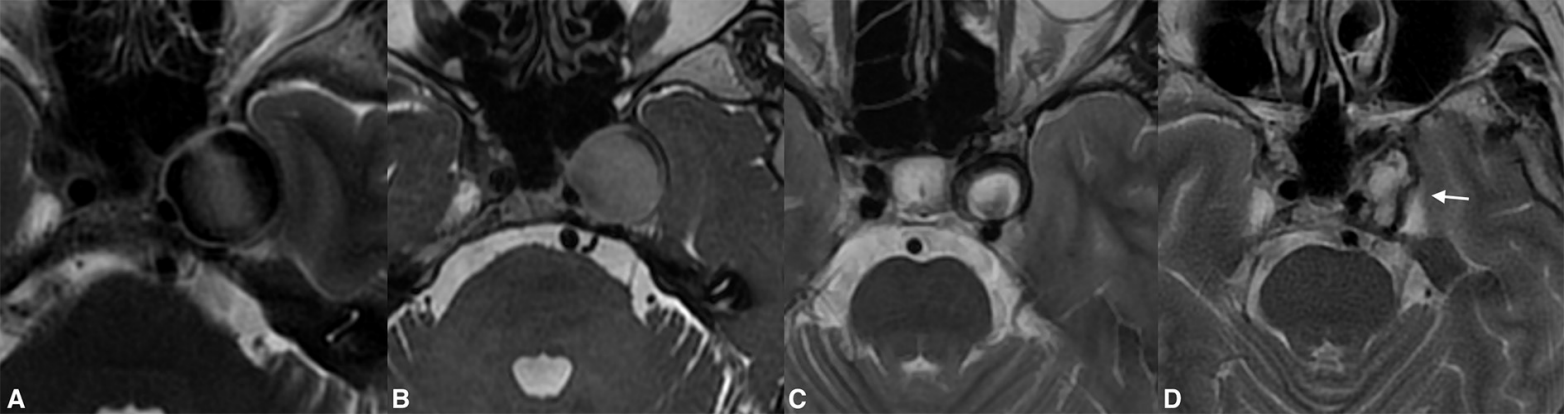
Giant extradural ICA (A) aneurysm demonstrating significant shrinkage following flow diversion. Despite the complete thrombosis of the aneurysm found on the first radiological follow-up, there were neglectable changes in the aneurysmal volume (B). One year post-treatment, the aneurysm shrinkage was visible on the MRI (C). The complete resolution of the aneurysm-induced compression of the left temporal lobe and the significantly diminished volume were present on long-term follow-up [(D), arrow].
